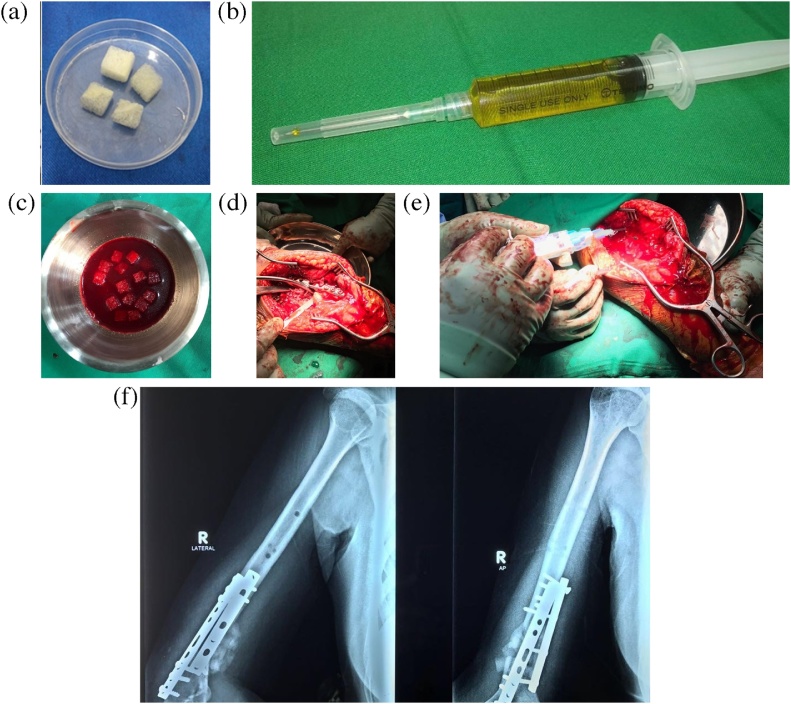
(a) Freeze-dried cancellous bone allograft produced by our tissue bank; (b) PRP; (c) the mixture of cancellous bone allograft, PRP and aspirated bone marrow; (d) application of the mixture to the defect site; (e) Sealing with fibrin glue; (f) Postoperative x-ray (source: internal documentation).
